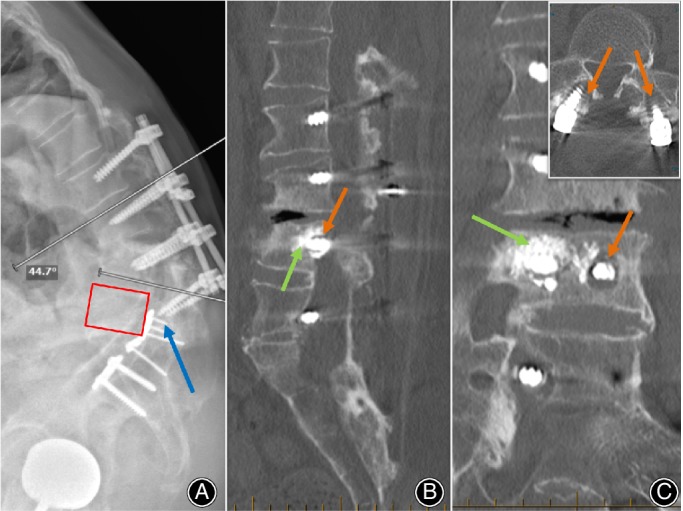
(A) Standing full‐body EOS scan; red box showing position of L_5_ vertebral body; blue arrow showing pull‐out of L_5_ pedicle screws. The acute kyphotic angle at L_3/4_ due to the construct failure was approximately 45^o^. (B) Sagittal CT with L_2_‐L_5_ pedicle screw construct with L_4_ cement‐augmented pedicle screws in vertebral body (green arrow). (C) L_4_ cement‐augmented pedicle screws (green arrow) on coronal CT view. Inset: L_5_ Proximal screw toggle with halo around pedicle screw and loosening of construct. Orange arrows indicate peri‐screw haloing due to cranial‐caudal toggling.
